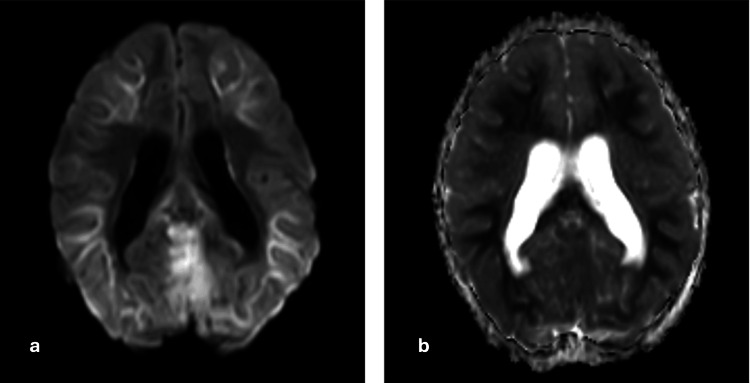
MRI brain axial sections (a) DWI and (b) ADC sequences showing diffusion restriction in the subcortical white matter of bilateral cerebral hemispheres. MRI: magnetic resonance imaging; DWI: diffusion-weighted imaging; ADC: apparent diffusion coefficient
